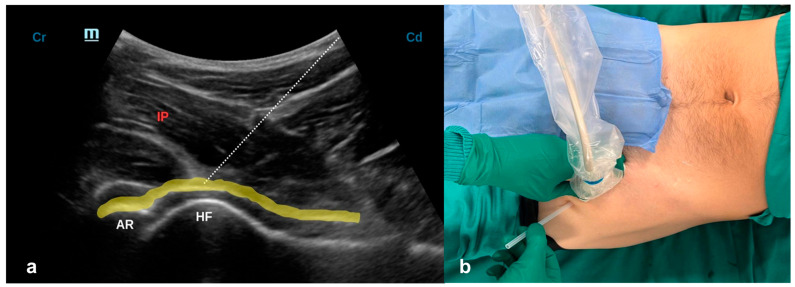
(a) Ultrasound scan for A-Peng block. The needle tip (dashed line) is advanced toward the pericapsular plane. The yellow line indicates the ideal distribution of the LA along the entire pericapsular plane, deep to the IP. Cr = cranial; Cd = caudal; AR = acetabular rim; HF = femoral head; IP = iliopsoas muscle. (b) Probe positioning for the A-Peng block. With the patient in the supine position, the convex probe is placed in a plane parallel to the long axis of the femoral neck. An echogenic needle is inserted in-plane, using a caudo-cranial approach, from lateral to medial.
